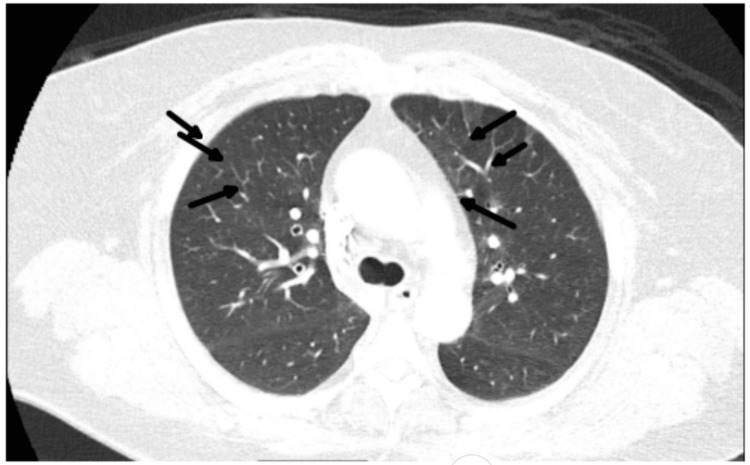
Chest CT angiography on hospital day 1: excluded pulmonary embolism but showed right middle lobe atelectasis and bilateral patchy parenchymal opacities suggestive of inflammatory changes. CT: computed tomography
